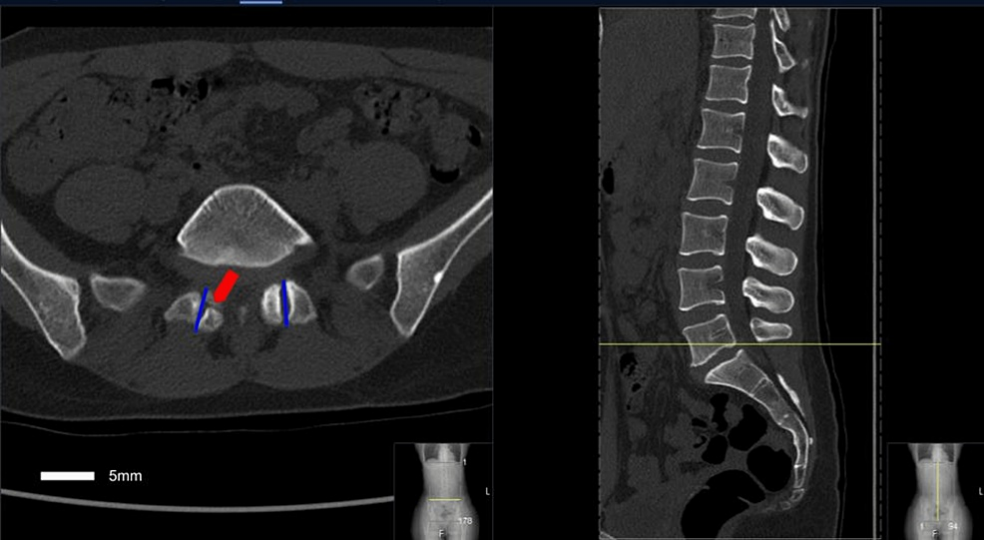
An axial (left) and sagittal (right) CT scan of the lumbar spine at the L5-S1 level Note the pars defect on the right (red arrow), as well as the sagittal orientation of the L5-S1 facet joint line (blue lines).
